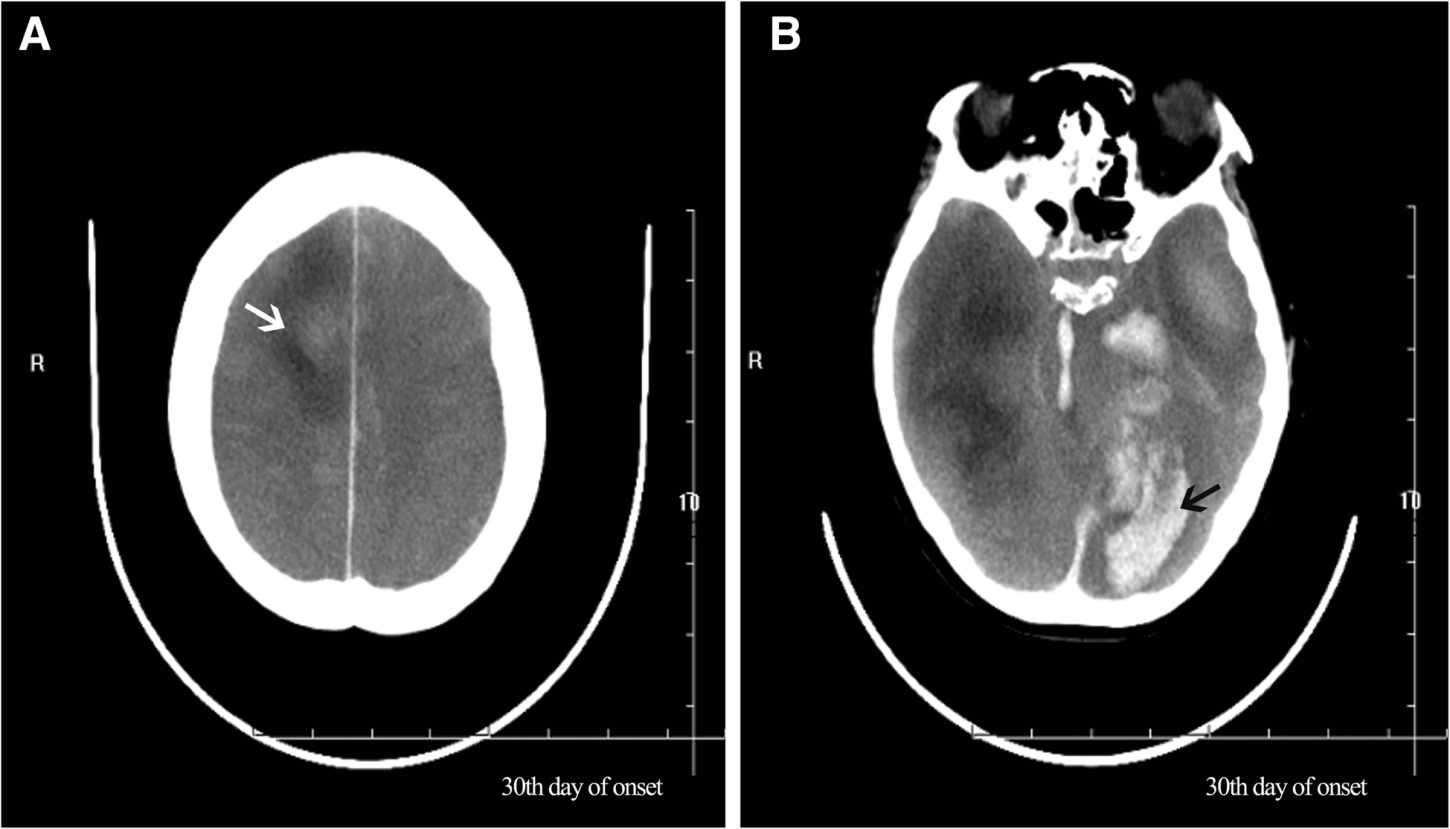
a, b A repeated CT scan of the brain showed the following: 1. The hemorrhages and densities (white arrow) in the right frontal lobe and left temporal lobe were decreased; 2. A new massive brain hemorrhage (black arrow) can be seen in the left occipitoparietal and left basal ganglia, and hematoma broken into side ventricles; 3. The midline deviated from the falx cerebri to the right side; 4. Subarachnoid hemorrhage was apparent
